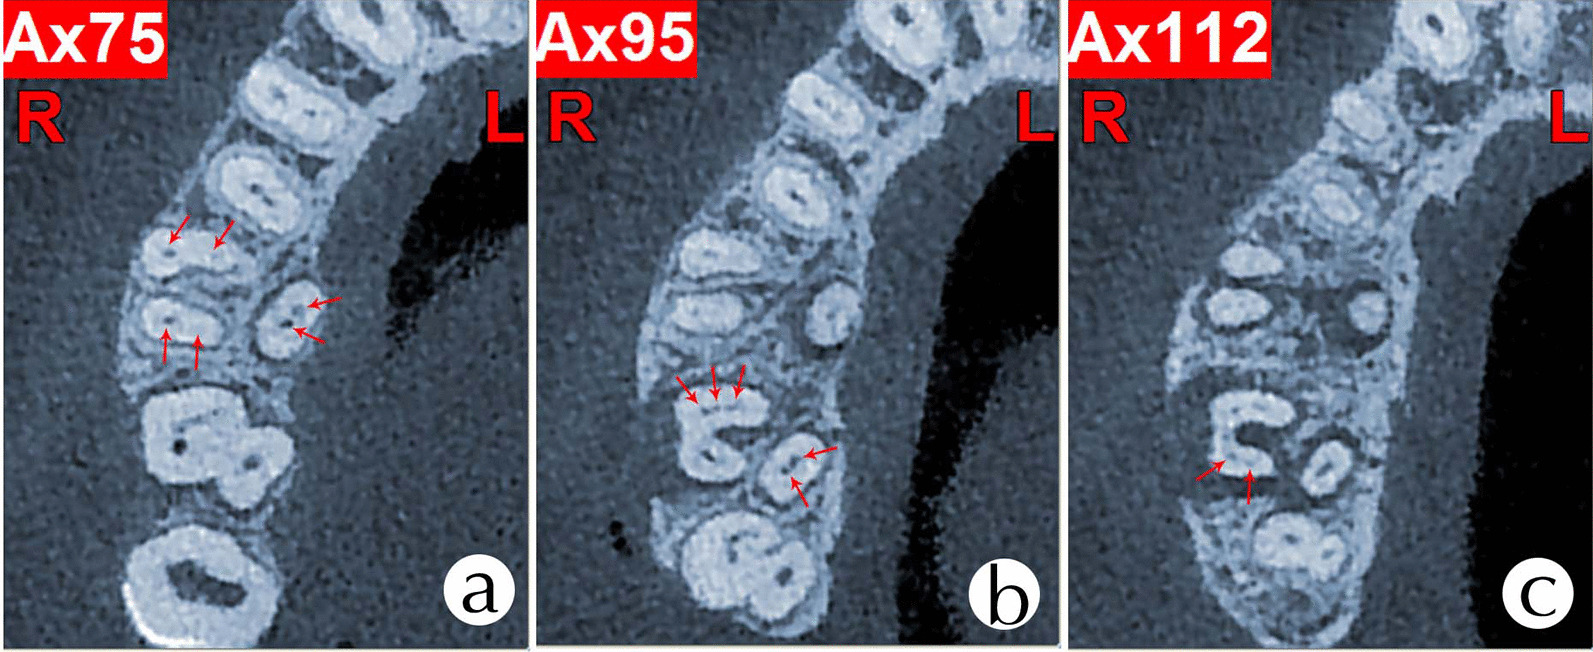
CBCT axial slice showing the a coronal, b middle, and c apical thirds of teeth #16 and #17: Arrow showing the a six root canals of tooth#16 and three mesiobuccal root canals. b Two palatal root canals of tooth #17. c Two distobuccal root canals of tooth #17
